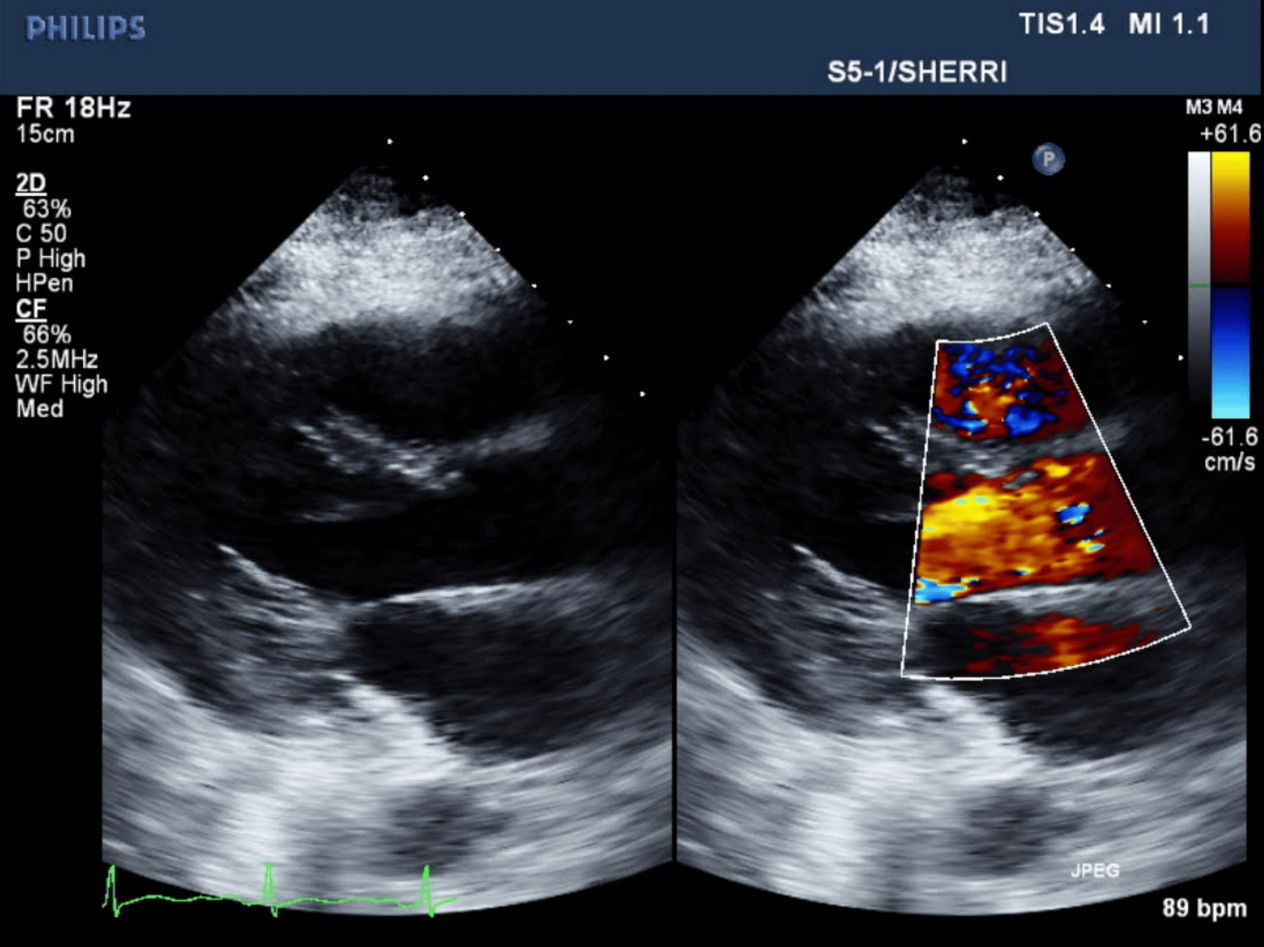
Second transthoracic echocardiography since admission with mitral valve visualization without any vegetation
